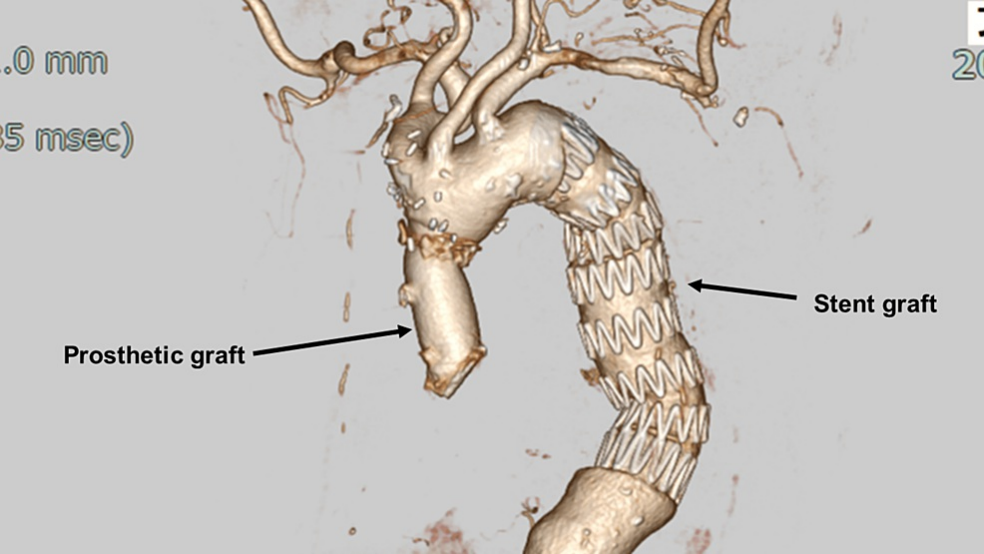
Postoperative computed tomography image A postoperative computed tomography scan revealed no dissection at the aortic arch.
